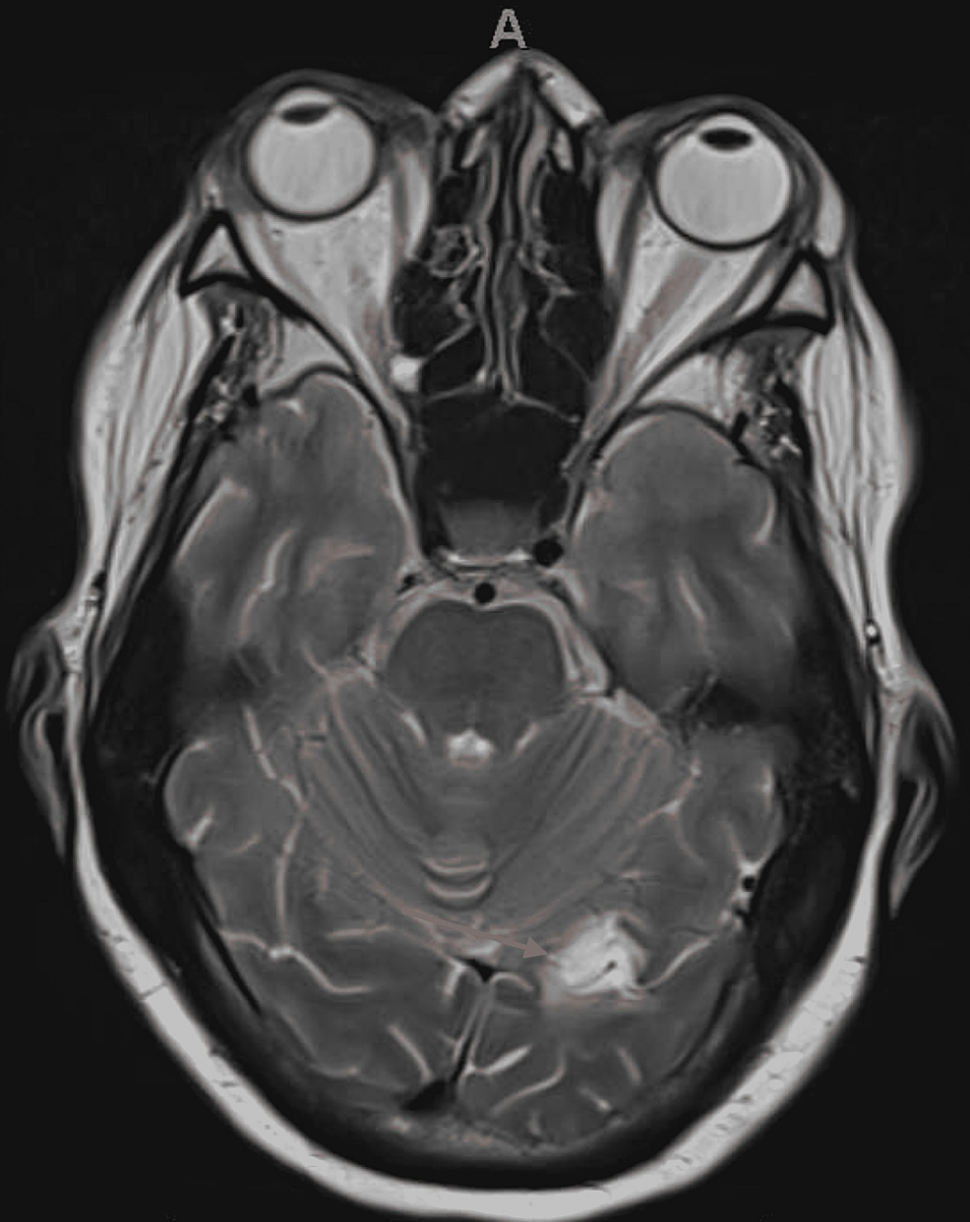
MRI of the brain - image 2 The red arrow is showing the chronic left occipital lobe lesion on this T2-weighted MRI image MRI: magnetic resonance imaging
